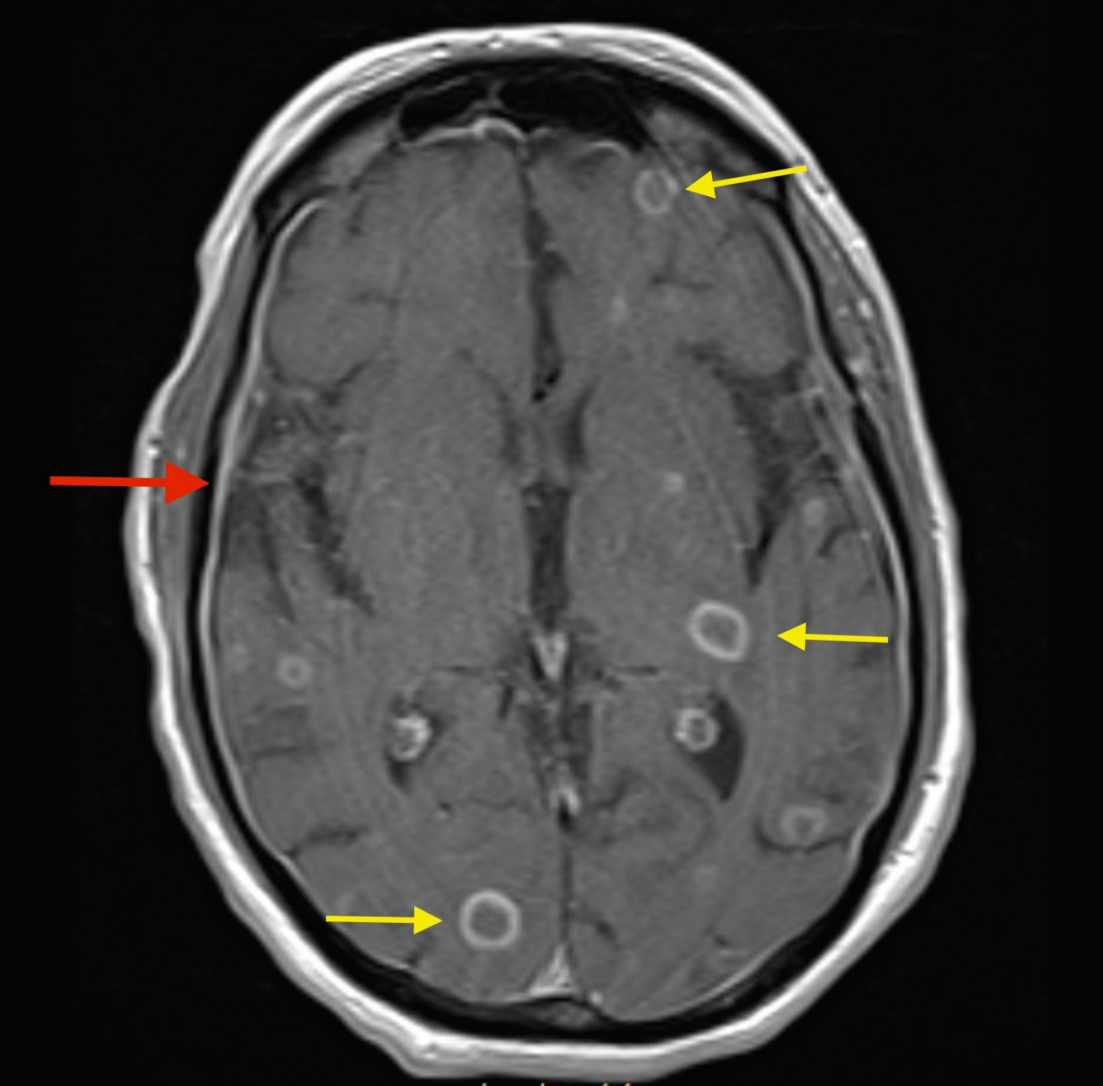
Magnetic resonance imaging (MRI) of the brain with contrast depicting meningeal enhancement (red arrow) with ring-enhancing lesions involving multiple lobes (yellow arrows).
